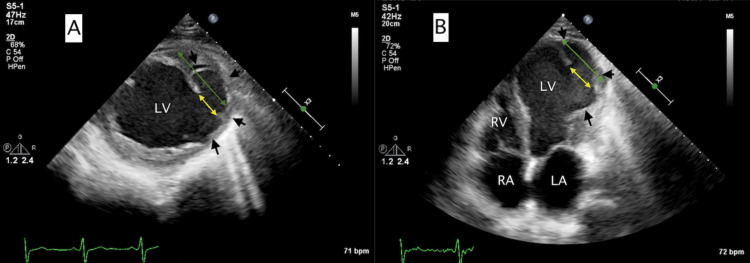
Transthoracic echocardiogram with pseudoaneurysm Echocardiogram unveiled a severely reduced ejection fraction of less than 15% and a left ventricular pseudoaneurysm upon the mid-anterolateral wall with a narrow neck of a max diameter of 2 cm (yellow arrows), corresponding to the borders of the ruptured wall, compared to a wider aneurysmal sac size of approximately 6-7 cm (green arrows). A: Two-dimensional transthoracic echocardiogram left ventricular short axis view with left ventricular pseudoaneurysm (black arrows). B: Two-dimensional transthoracic echocardiogram four-chamber view with left ventricular pseudoaneurysm (black arrows) LV: left ventricle, LA: left atrium, RV: right ventricle, RA: right atrium
